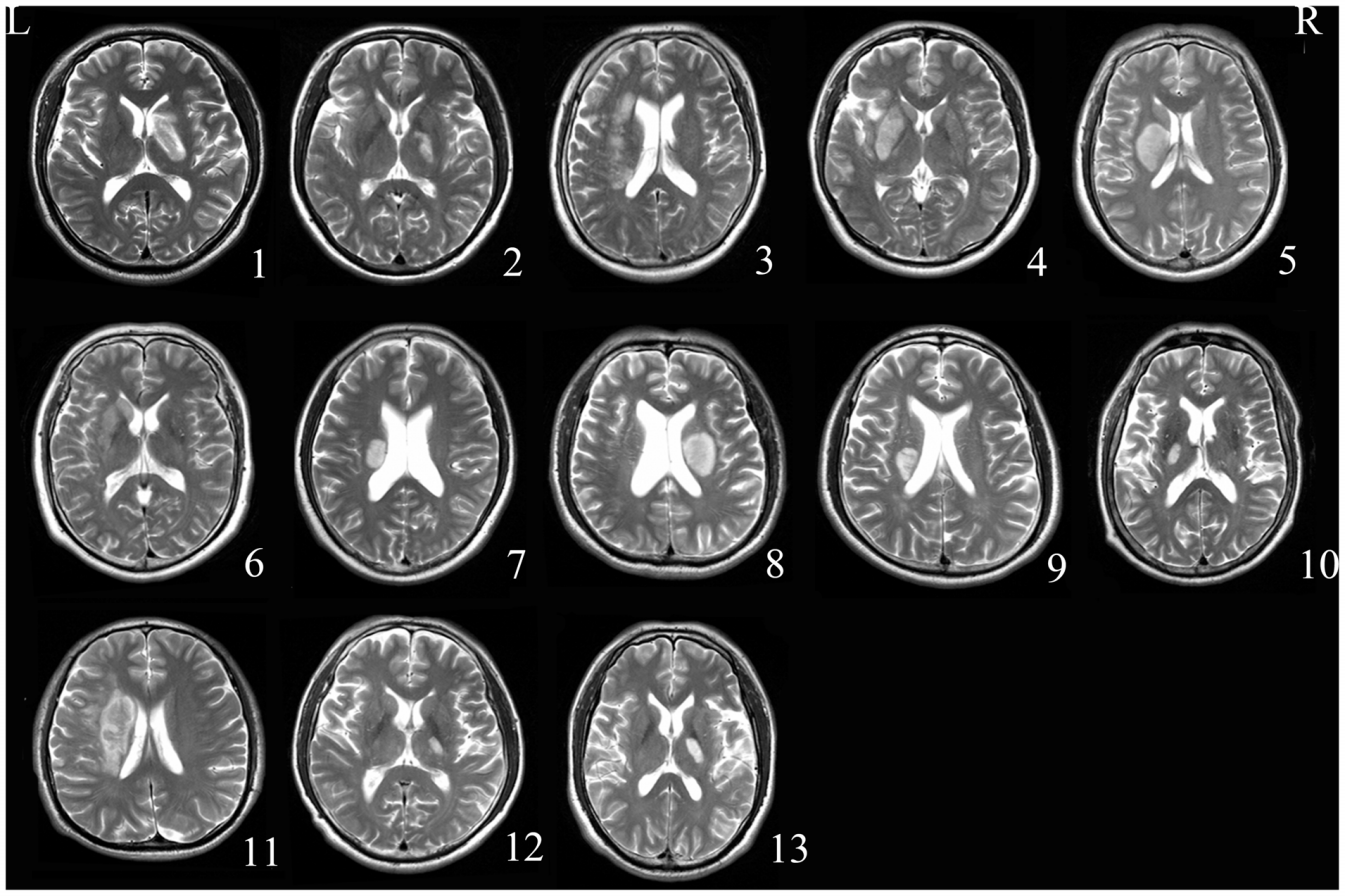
Lesion locations of stroke patients. The lesions are shown on axial slices of the T2-weighted images. Patient numbers correspond with those in Table 1. L, left and R, right.
